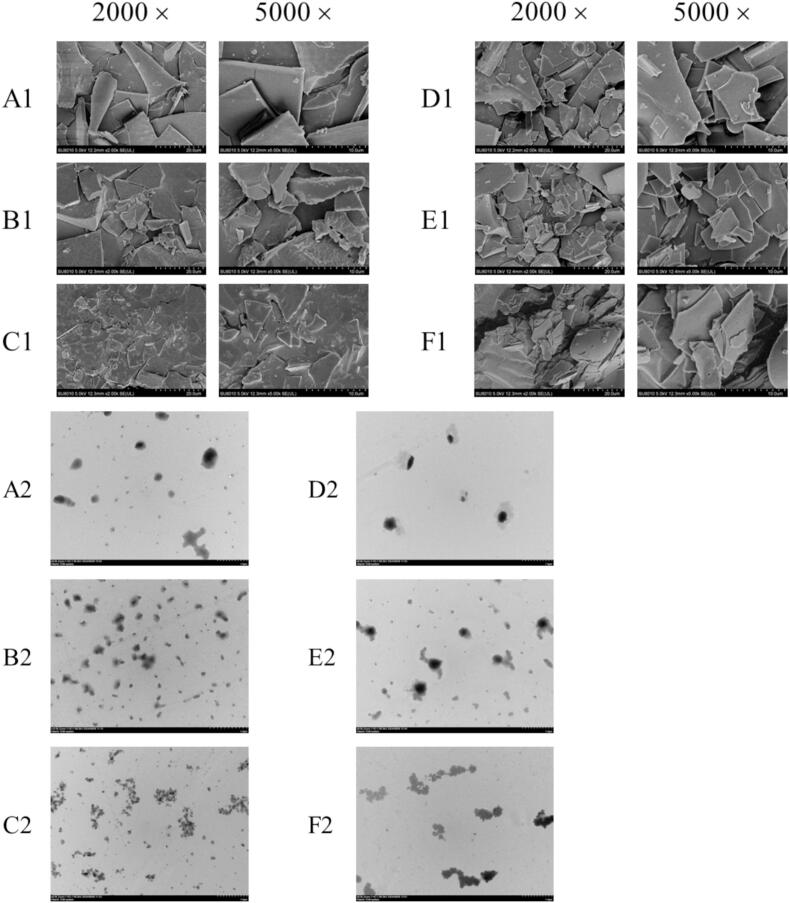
SEM (A1-F1) and TEM (A2-F2) images of CH, crosslinked CH and glycated CH before and after ultrasound treatment. Difference lowercase letters indicate a significant difference at *p* < 0.05.
